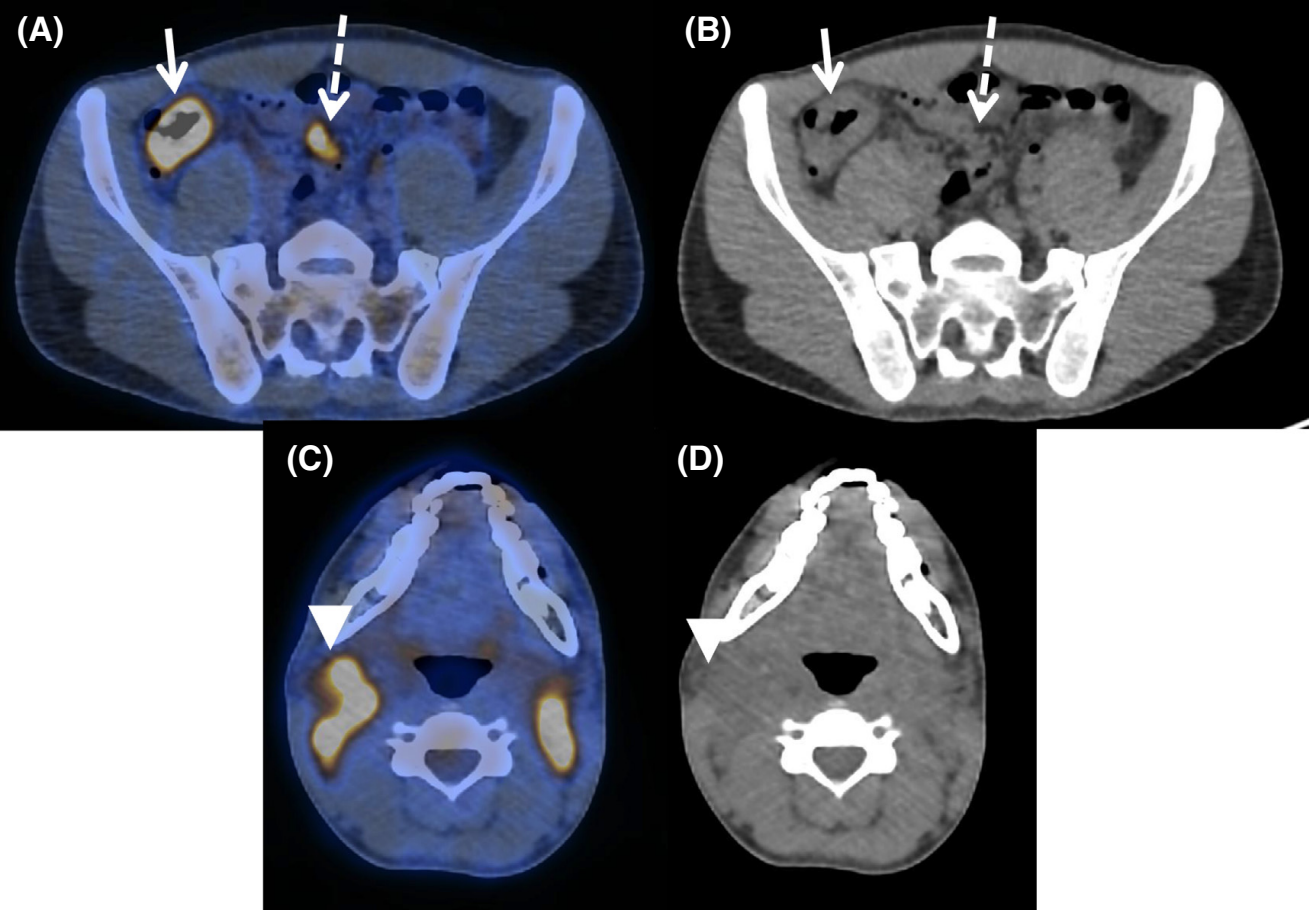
(A, C) ^18^F‐FDG PET/CT axial fused and (B, D) CT scan image of initial Hodgkin lymphoma staging. (A, B) Arrow shows colon tuberculosis localization (SUVmax 10.9), associated with mesenteric TB lymph nodes (dotted line) (SUVmax 6.6). (C, D) Arrowhead shows cervical lymph nodes of Hodgkin lymphoma, SUVmax 10.7. Measurement of SUVmax is inadequate to differentiate TB and HL disease.
